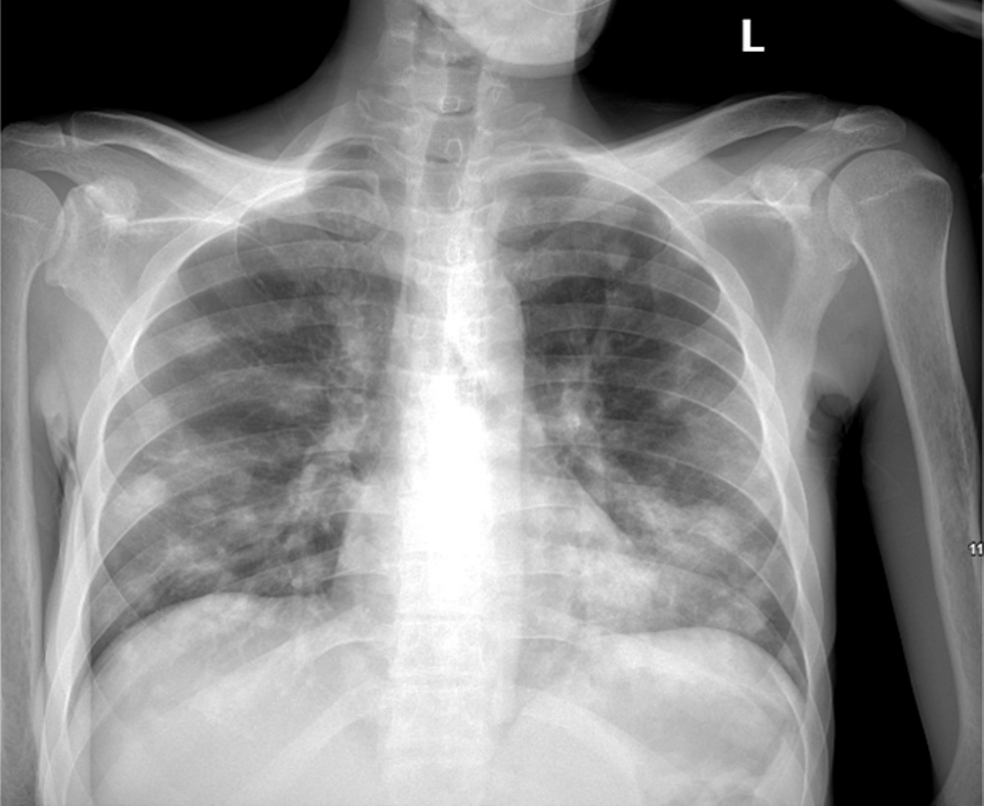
Chest X-ray showing bilateral patchy opacities.
